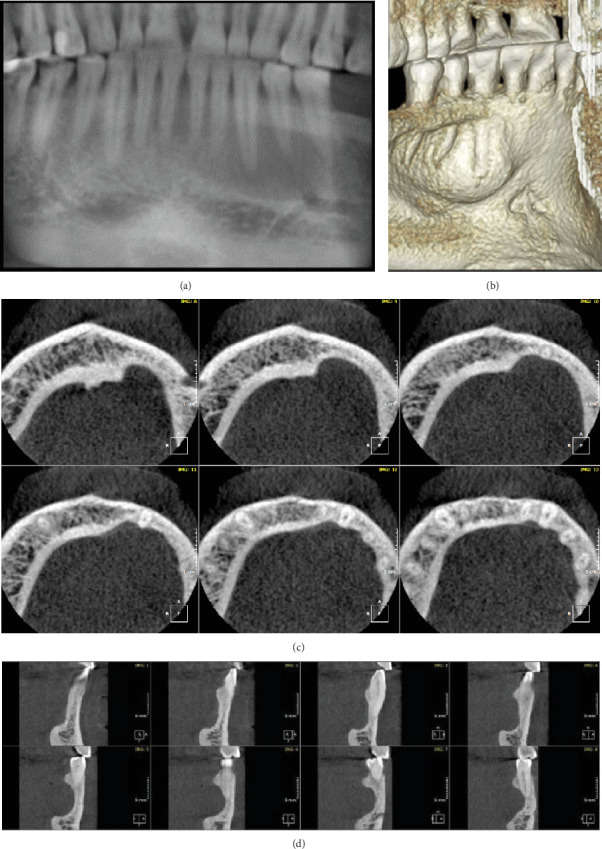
Case 4. CBCT scan with the following views: (a) reconstructed panoramic, (b) 3D rendering reformatting image represents of the extends and area of the bone defect from the inner surface of the mouth, and (c) serial axial cuts representing the curvature and shape of the lingual depression from deep at the bottom to saucering/scalloping. (d) Corrected coronal sectional images show the area of appreciated lingual defect at the left body of the mandible.
